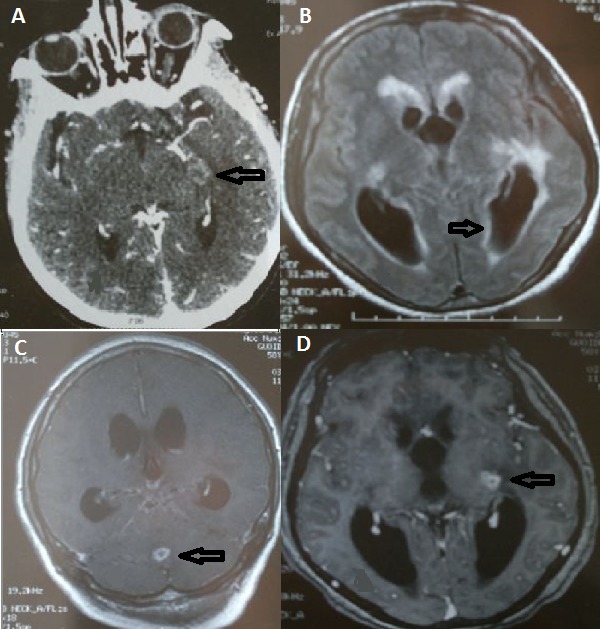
Brain imaging signs of tuberculous meningoenpahlitis: CT scan and MRI: A) temporal abscess in brain CT scan after injection of contrast; B) dilated ventricles (hydrocephalus) in magnetic resonance imaging (MRI); C) Vvrmian abscess in MRI T1 weighted; D) temporal abscess in MRI T1 weighted
